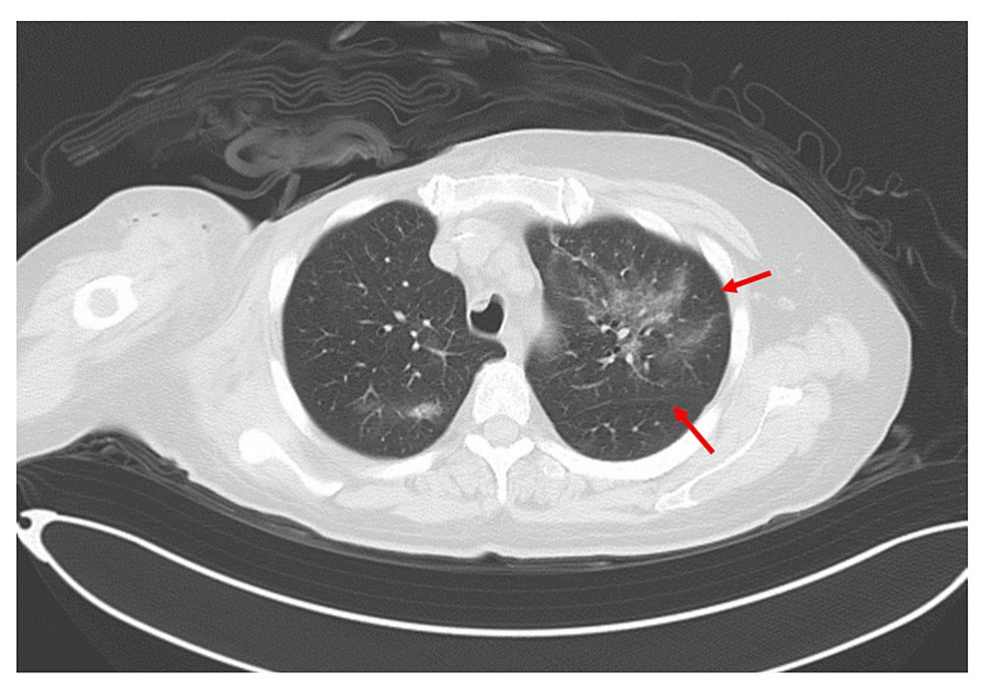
CT image of the patient on day 289. Ground-glass opacities were noted within the S1-2 segments of the left upper pulmonary lobe (arrows).
